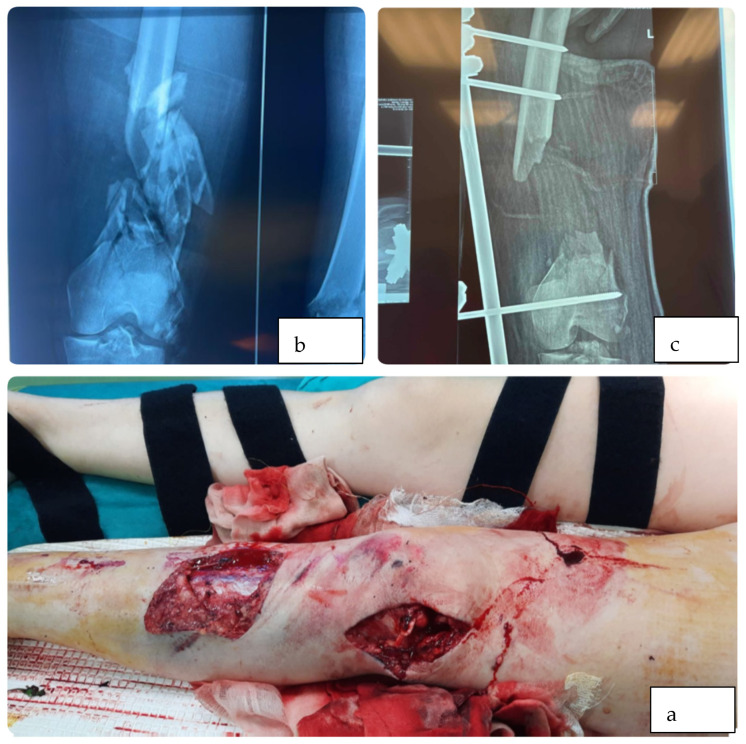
Case 3 (Part 1): Initial presentation and damage-control phase in a 50-year-old female following high-energy trauma. (a) Clinical presentation of the massive Gustilo–Anderson type IIIB open fracture in June 2022. (b) Initial anteroposterior radiograph revealing the severe comminuted distal femoral fracture with massive segmental bone loss in June 2022. (c) Radiograph demonstrating the first-line stabilization utilizing a joint-spanning external fixator to allow for soft-tissue management in July 2022.
